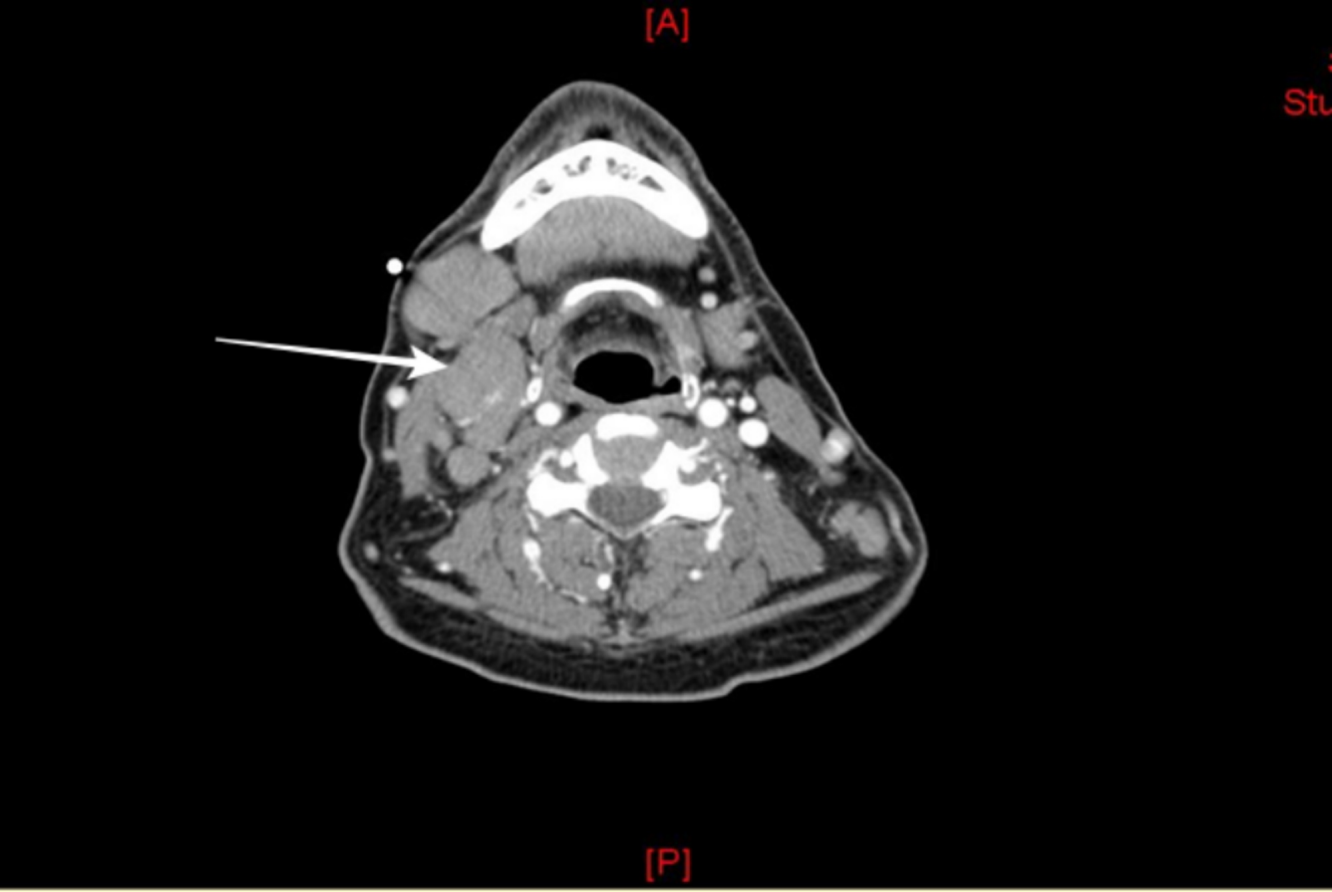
Computed tomography of cervical soft tissue Diffusely enlarged lymph nodes; the largest, most superficial, and most amenable to
